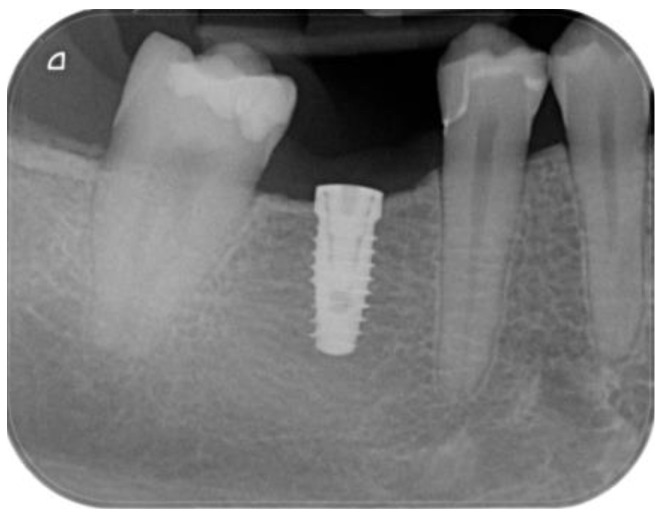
Intra-oral control X-ray.
